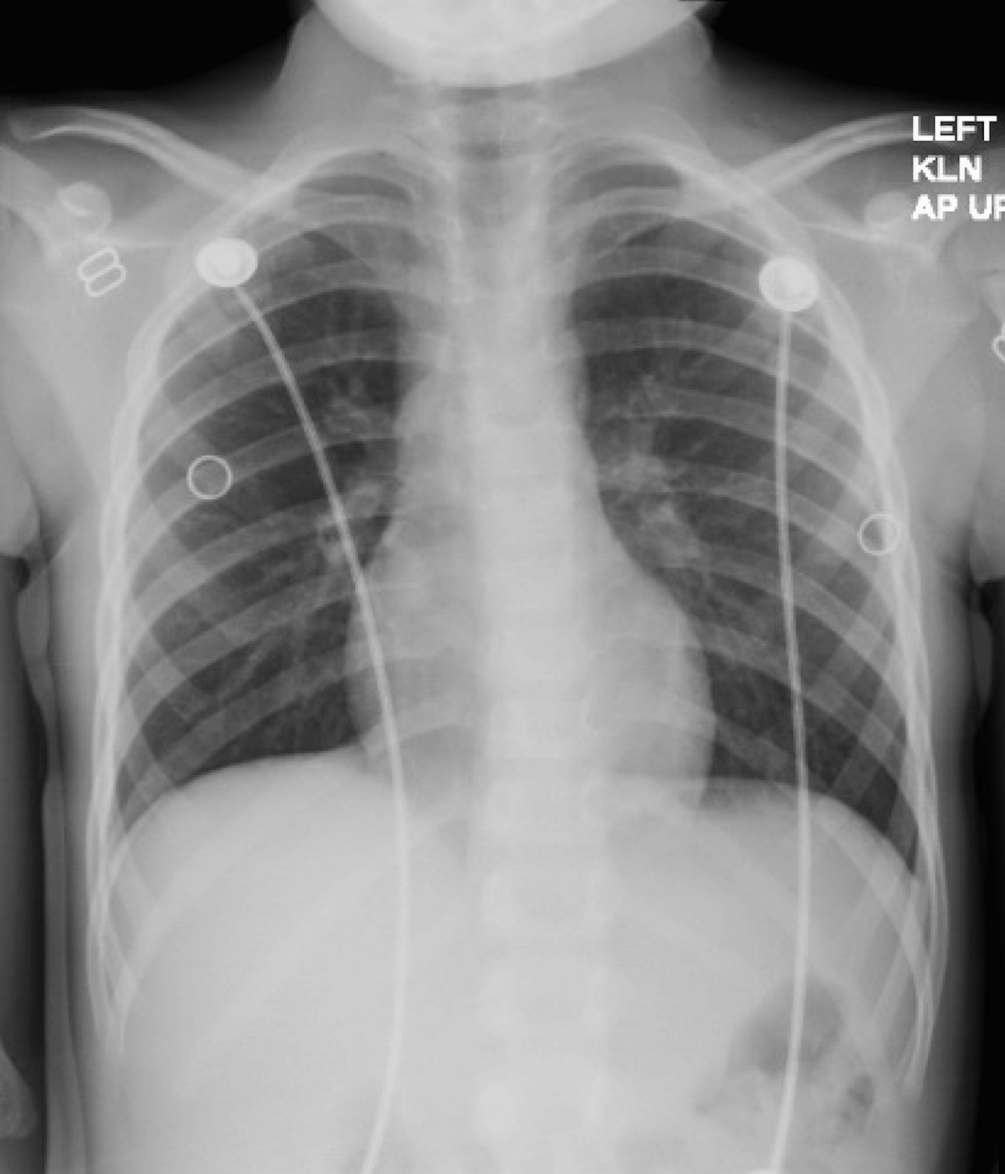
Patient's chest radiograph
